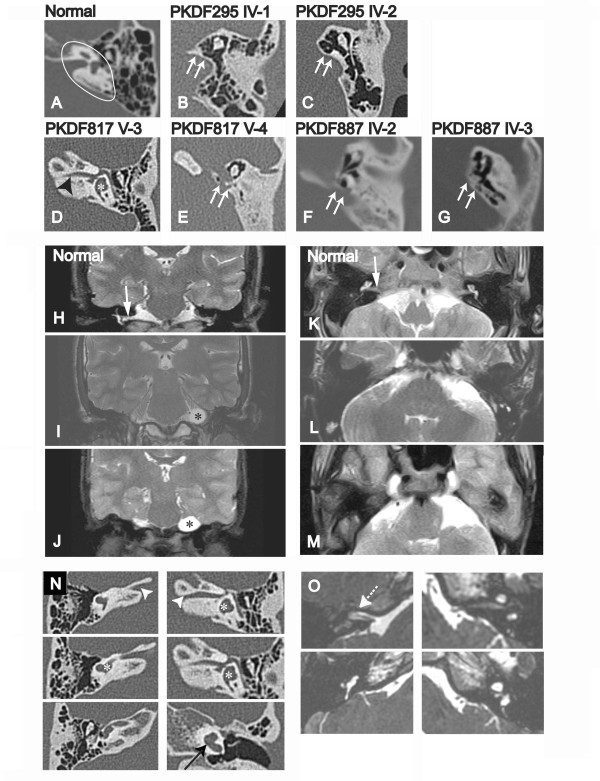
Radiological images from affected individuals with *FGF3 *homozygous mutations. Images (A through G) are axial temporal bone CT sections. (A) Inner ear structures (Internal auditory canal (IAC), cochlea, and vestibule) in petrous bone are clearly seen (white ellipsoid) in a normal subject. (B, C, E, F, and G) Complete labyrinthine aplasia with a flat medial wall (white arrows) of the middle ear cavity. (D) A narrow IAC (black arrowhead), posterior semicircular canal and vestibule (white asterisk) are visible. Images (H-J and K-M) are coronal and axial sections, respectively, of T2-weighted MR images (T2WI) of the cerebellopontine angle region. Affected individuals, PKDF295 IV-2 (I and L) and PKDF887 IV-3 (J and M) have a presumed subarachnoid cyst (black asterisk). The cochleovestibular nerve and inner ear structures (white arrow) detected in a normal subject (H and K) are not clearly seen in affected subjects (I, J and M). Images (N and O) are contiguous axial temporal bone CT sections and axial 3D-FIESTA MR images from PKDF817 V-3, respectively. (N) The osseous IAC (white arrowhead), vestibule (white asterisk), and 1-1/2 turns of the cochlea (black arrow) can be identified in the left ear. The vestibule and posterior semicircular canal are shown in the right ear. (O) The presence of a single cochleovestibular nerve (white dotted arrow) can be identified in the right side. Cochlea, vestibule and posterior semicircular canal are shown in the left ear.
